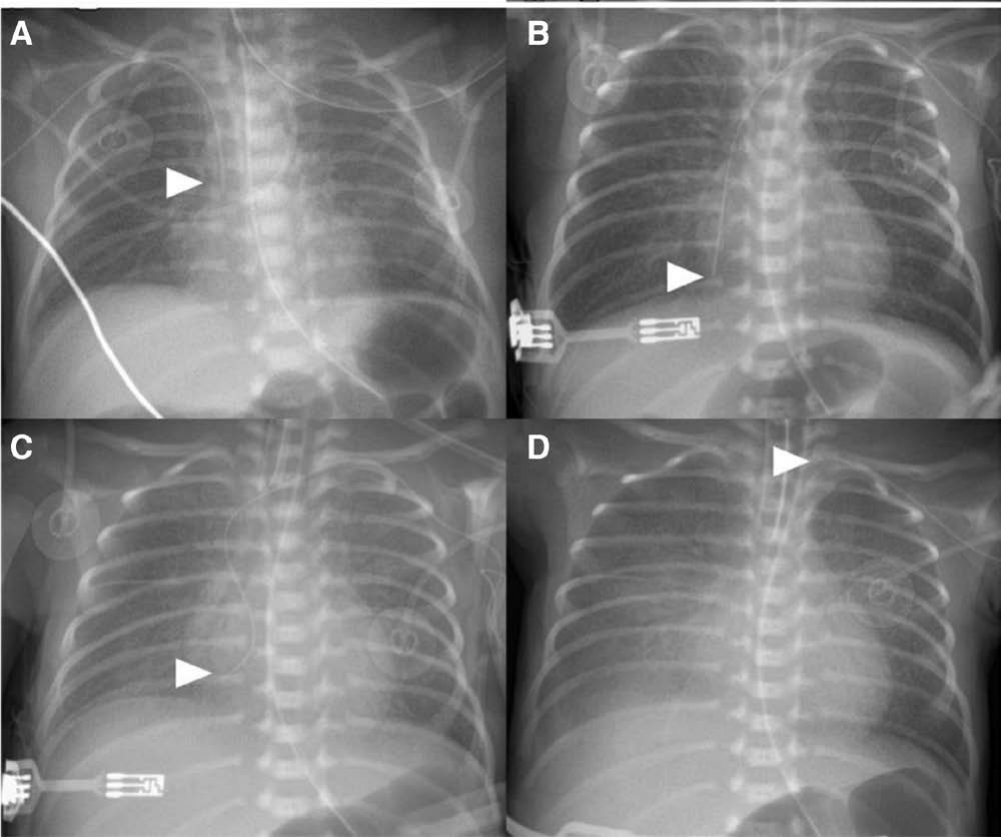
The distal tip of inserted PICC, in case 2 patient. Twelve hours after birth, on the second day of life, the PICC was inserted. The distal tip of the PICC line was identified at the outside of cardiac silhouette (A). The second PICC was inserted on the fiftth day of life. The chest radiography taken immediately after insertion showed the distal tip of PICC line was located inside cardiac silhouette (B), chest X-ray after sudden cardiopulmonary instability showed inappropriate position of the distal tip, curved and deep in the right atrium (C), the PICC was repositioned, the distal tip was placed outside of cardiac silhouette (D). The position of PICC tip was indicated by an arrow. PICC = peripherally inserted central catheter.
